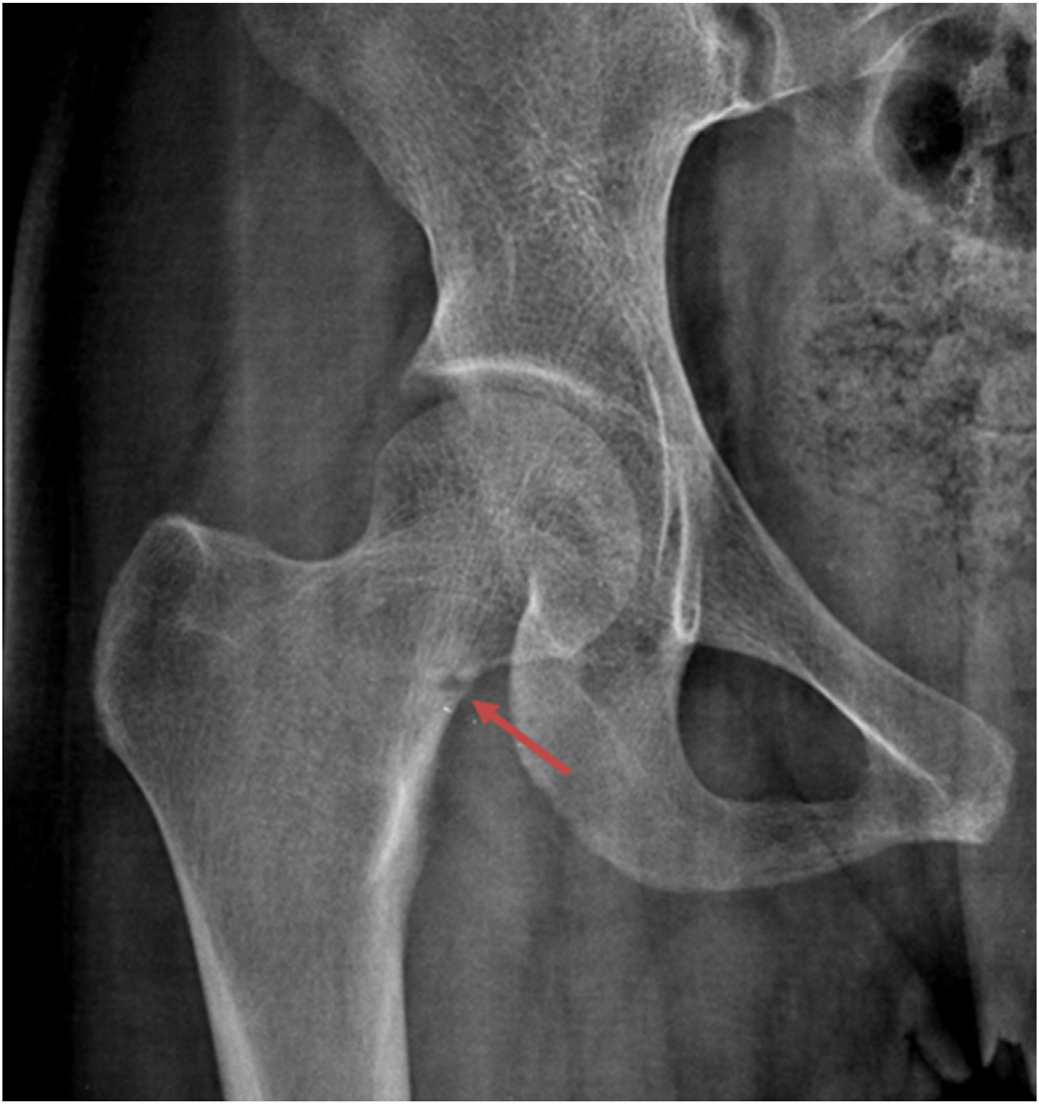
The radiograph depicts Looser zone involving the right medial femoral neck in a patient with osteomalacia (red arrow). Case courtesy of Dr. Maulik S Patel, Radiopaedia.org, rID: 16273. https://radiopaedia.org/cases/16273.
